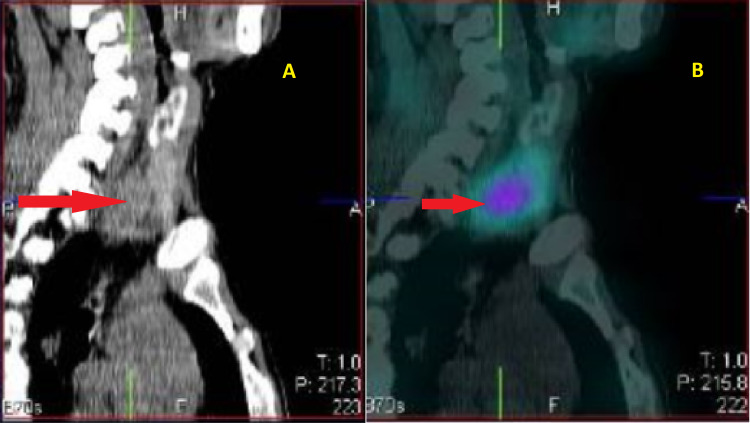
(A) Three-dimensional CT scan and (B) SPECT scintigraphy showing a right inferior parathyroid adenoma (arrow). SPECT, single-photon emission computed tomography.
